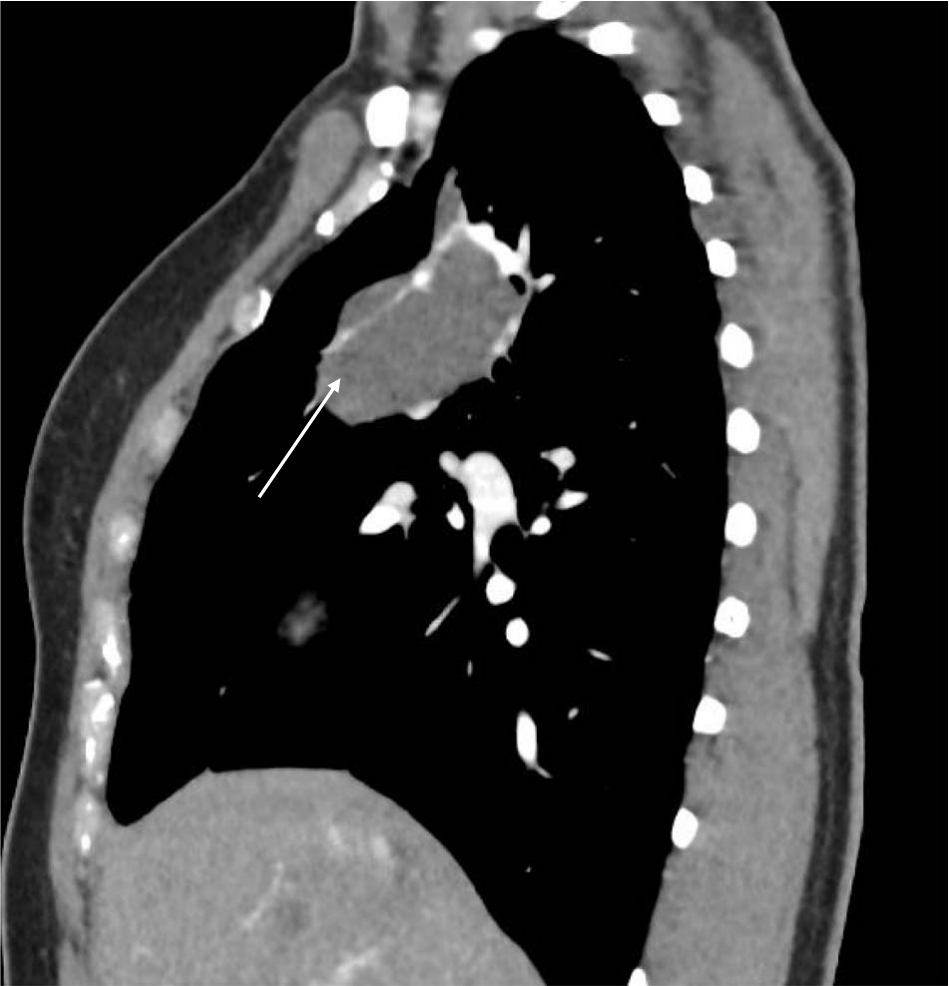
Sagittal CT scan demonstrating pulmonary sequestration in the right upper lung lobe (arrow).
